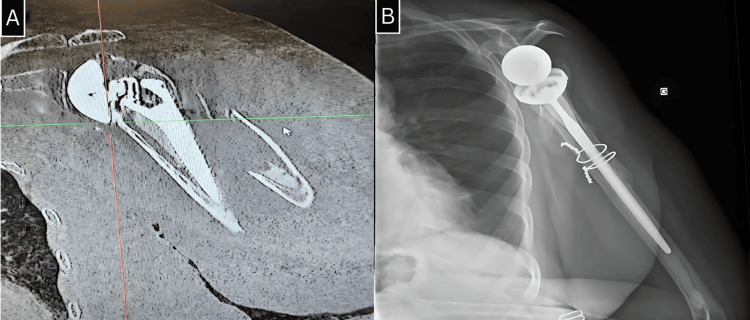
A: Frontal section of the CT scan image of a periprosthetic fracture of region 3 in the left shoulder. B: Frontal radiograph of the left shoulder shows the evolution after six months post-revision. CT: computed tomography
